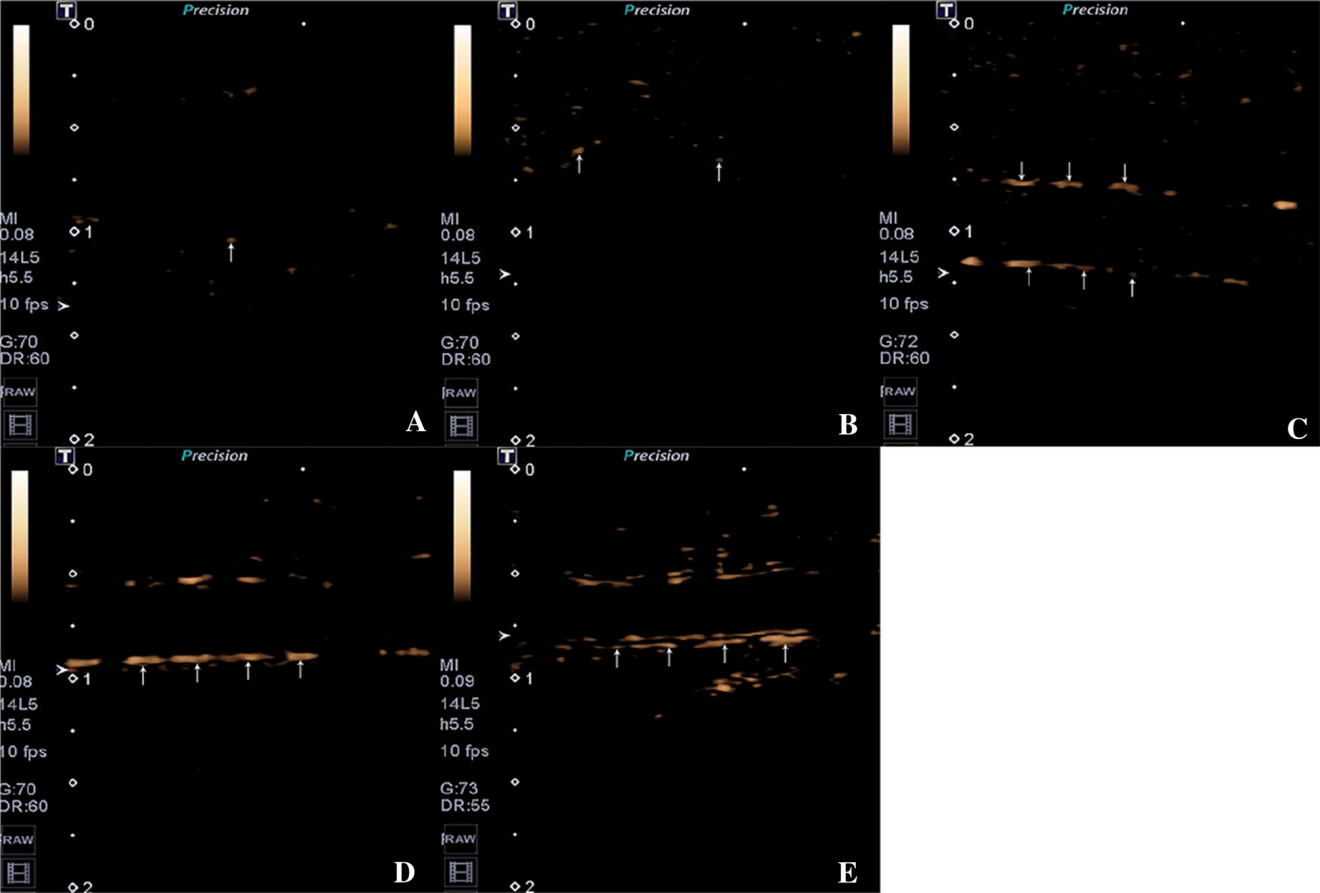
ICAM-1-targeted nano ultrasonic contrast images of abdominal aorta of experiment rabbits in different groups, five rabbits in each group. (A) Control group; (B) Week-4 group; (C) Week-8 group; (D) Week-12 group; (E) Week-16 group. The vascular wall was indicated by the arrows.
